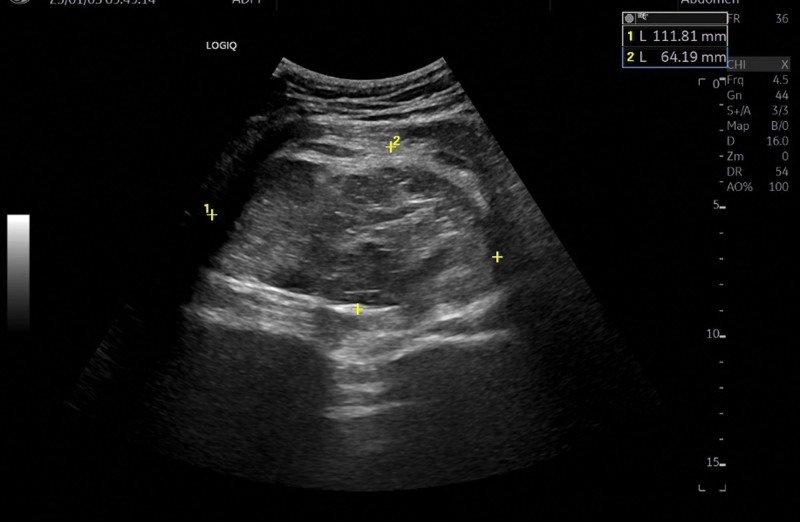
MSK-US. The MSK-US identified a 12.8 cm × 8.1 cm × 11.7 cm lobulated mass with regular margins and absence of vascular flow signals, suggestive of a solid lesion anterior to the piriformis muscle (recommended further investigation). MSK-US = musculoskeletal ultrasound.
